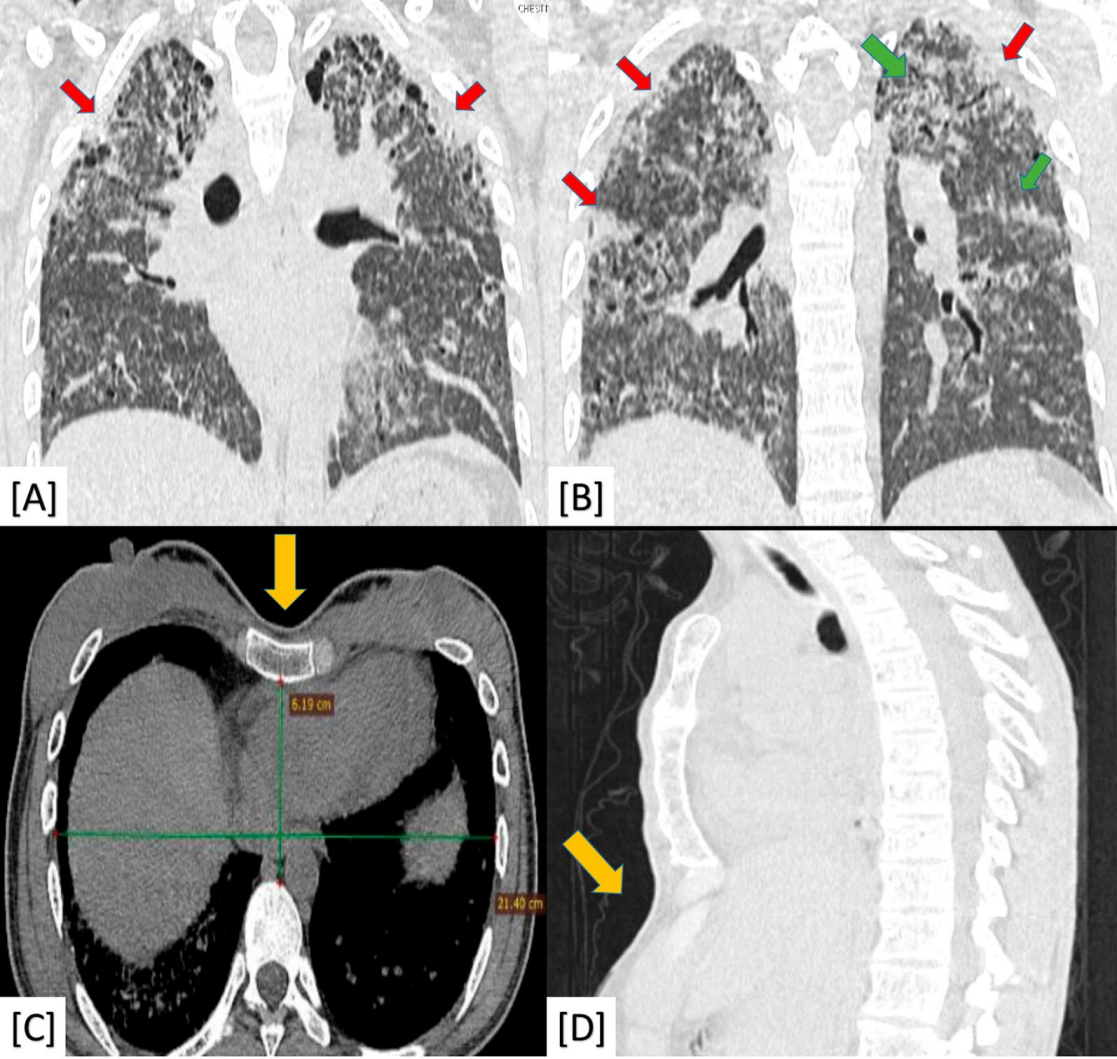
A 30-year-old female patient complaining of rapid progressive dyspnea. A, B Coronal chest CT (lung window) showing bilateral irregular pleural based (red arrows) and underlying parenchymal (green arrows) fibro-consolidative changes with sub-pleural cystic changes. C Axial chest CT (mediastinal window) showed a flat anterior chest wall ("platy-thorax" sign) with an evident reduction in AP diameter compared to transverse diameter. D Sagittal chest CT (lung window) showed also ("platy-thorax" sign). Diagnosis: Proved patient with pleuroparenchymal fibroelastosis (PPFE)
